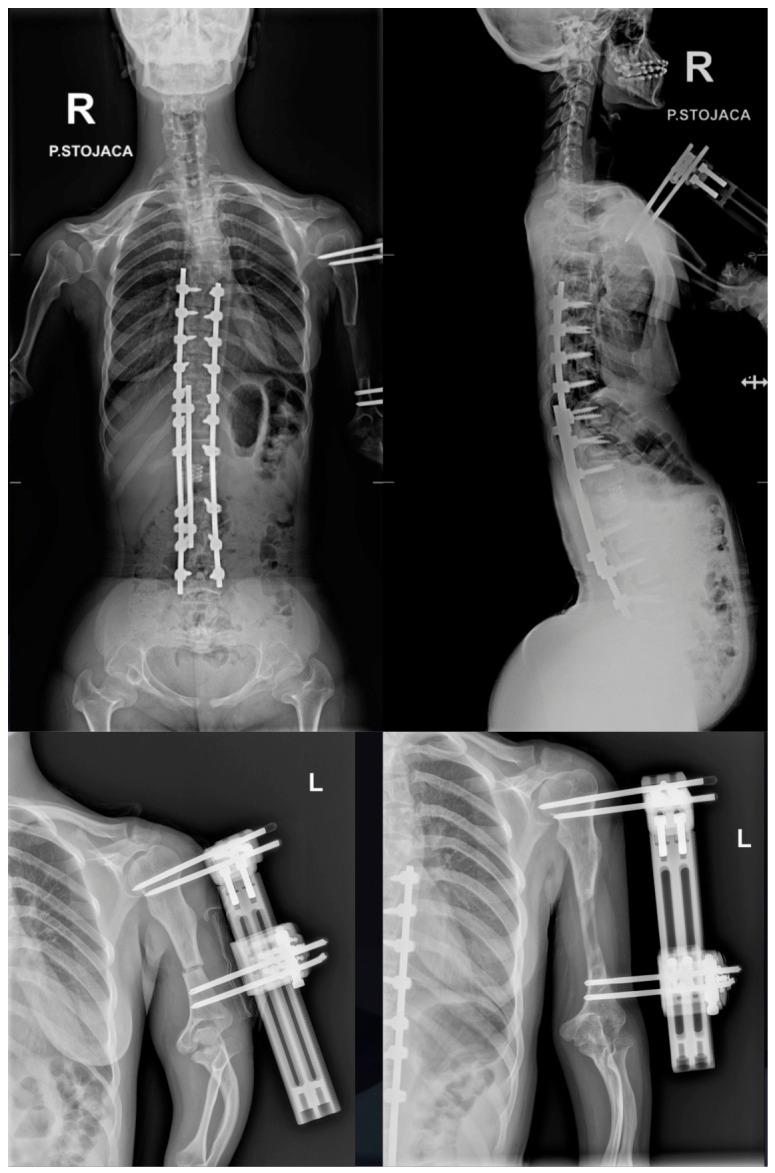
Radiographic images showing the left upper limb during the distraction phase of the lengthening procedure.
